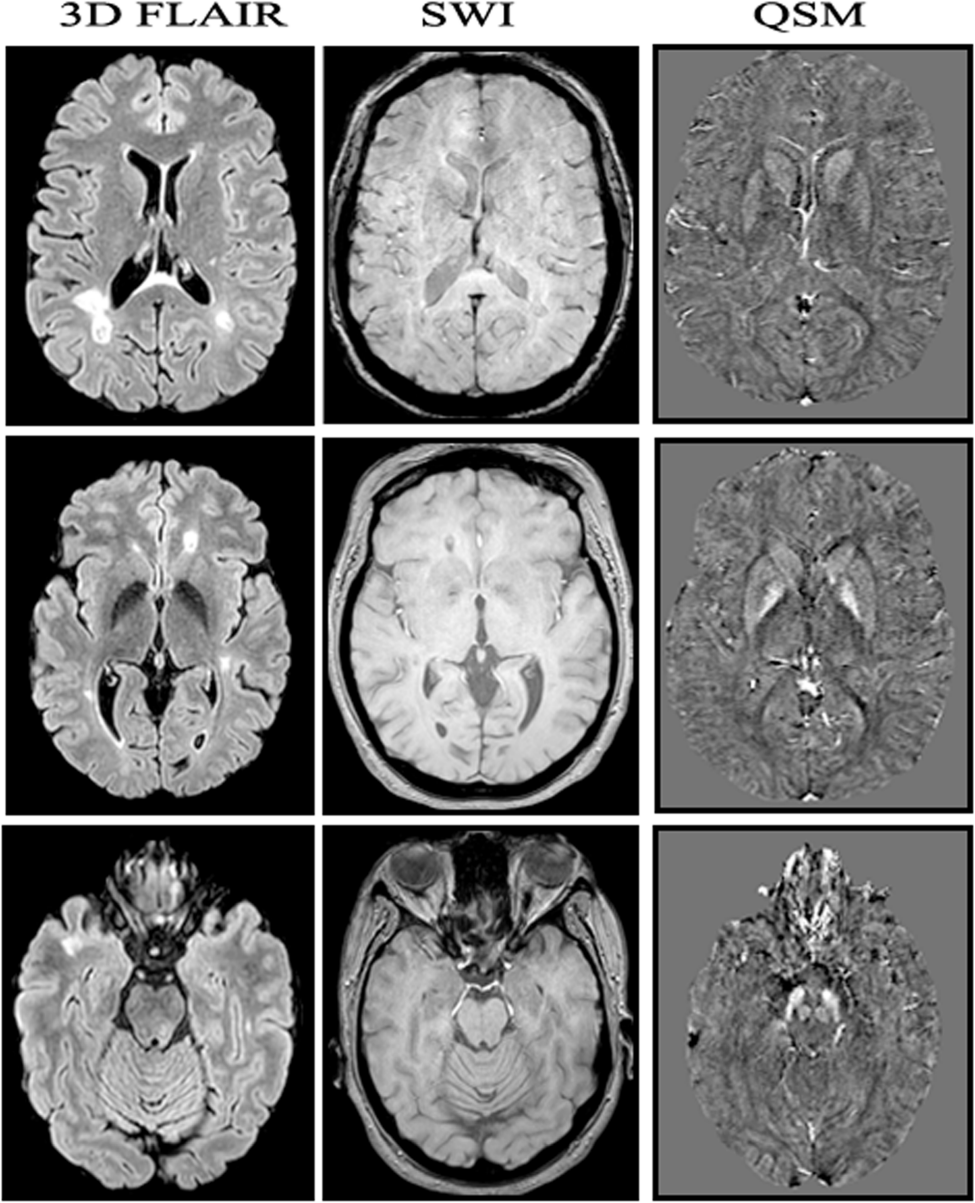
Magnetic resonance image of the brain of a 36-year-old lady diagnosed with relapsing-remitting multiple sclerosis. Three different imaging series of corresponding axial cuts are demonstrated, *i.e*., three-dimensional fluid-attenuation inversion recovery images, susceptibility-weighted images, and the reconstructed quantitative susceptibility maps
